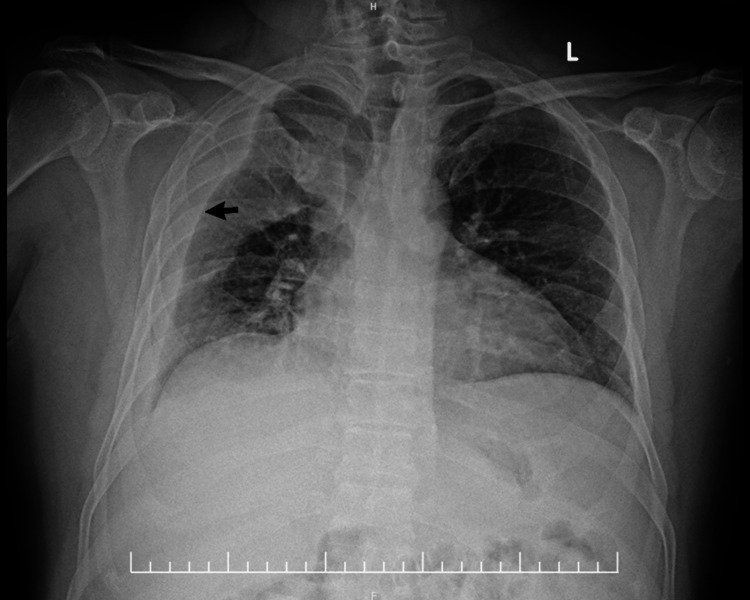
Postoperative chest radiograph demonstrating resolution. Posteroanterior chest radiograph taken after the patient underwent right percutaneous nephrolithotomy and double-J stenting. The image demonstrates near-complete resolution of the right-sided urinothorax and good expansion of the underlying lung parenchyma, confirming the successful definitive treatment.
